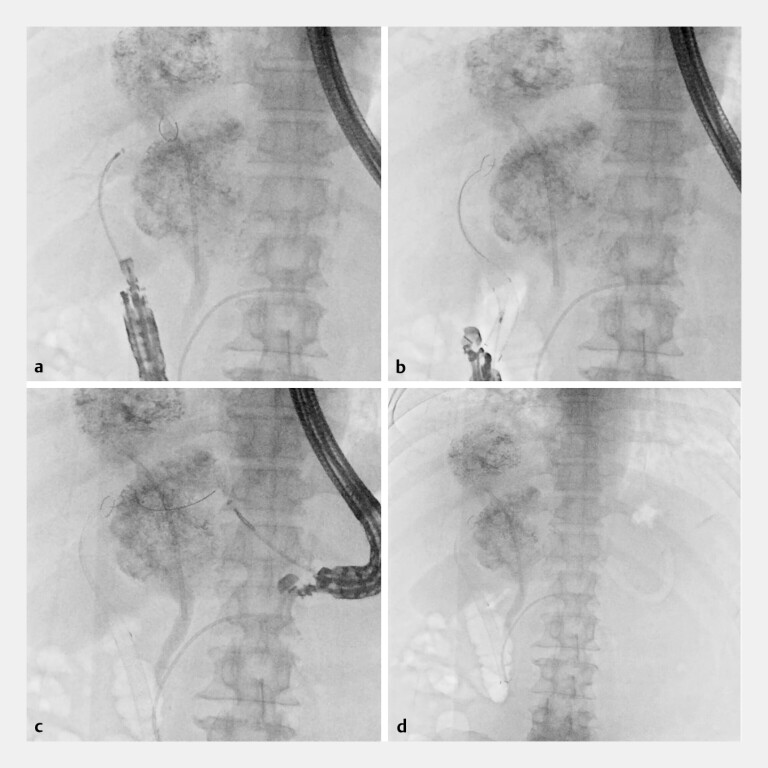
Fluoroscopic images during endoscopic ultrasound-guided hepaticoduodenostomy and hepaticogastrostomy.
